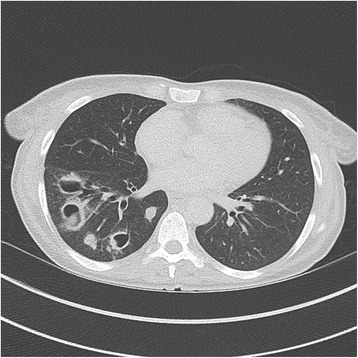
Thoracic CT scan showing a cavitary community-acquired pneumonia due to *A. pittii* with the three others cavitations and nodules of the right lower lobe, three days after admission
